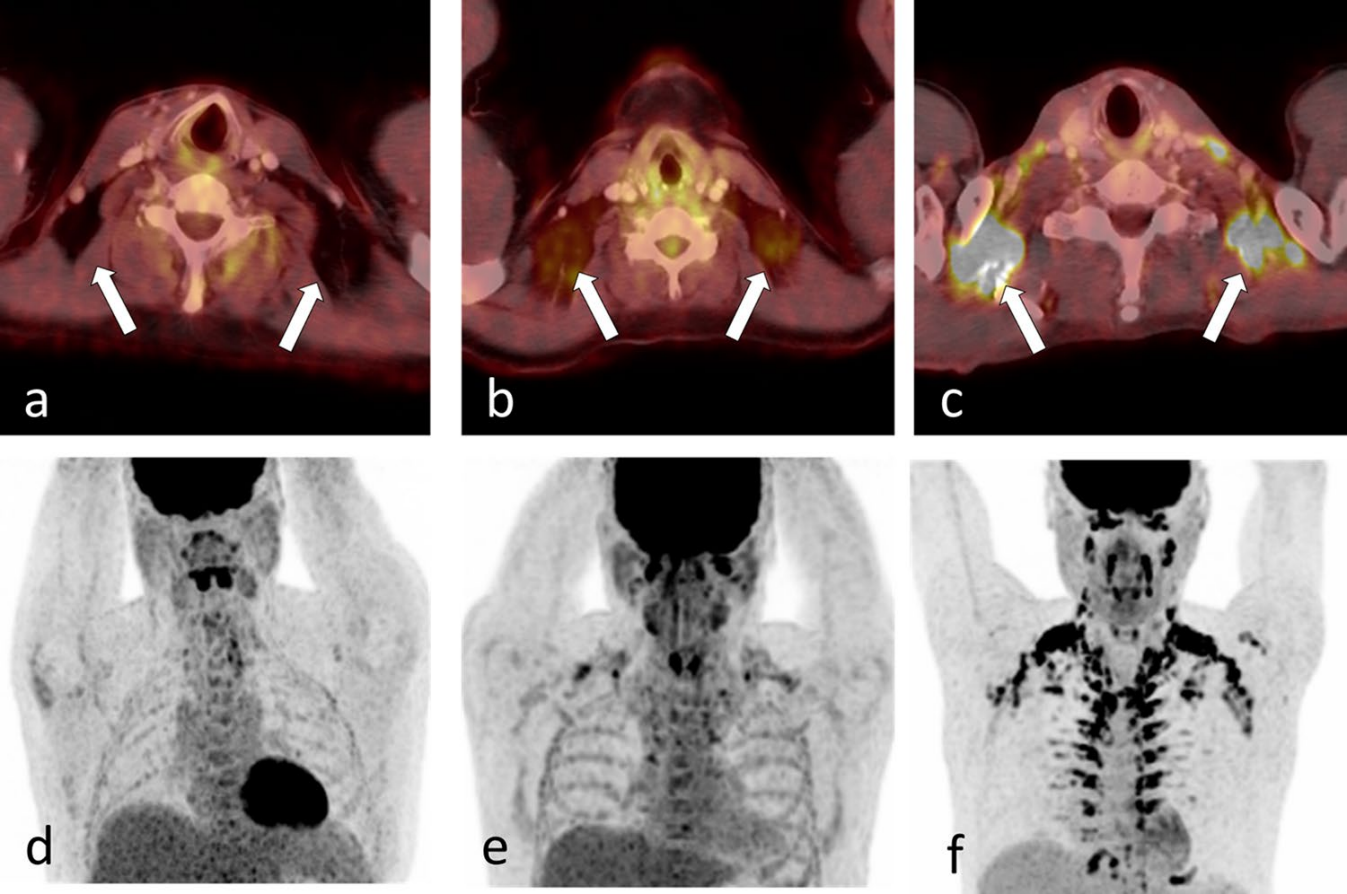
Categories of BAT activity. Axial fused PET/CT images (a–c) and maximum intensity projection of PET (d–f) of patient examples with no activity of brown adipose tissue (BAT, a,d), low BAT activity (b,e) and high BAT activity (c,f, see arrows).
